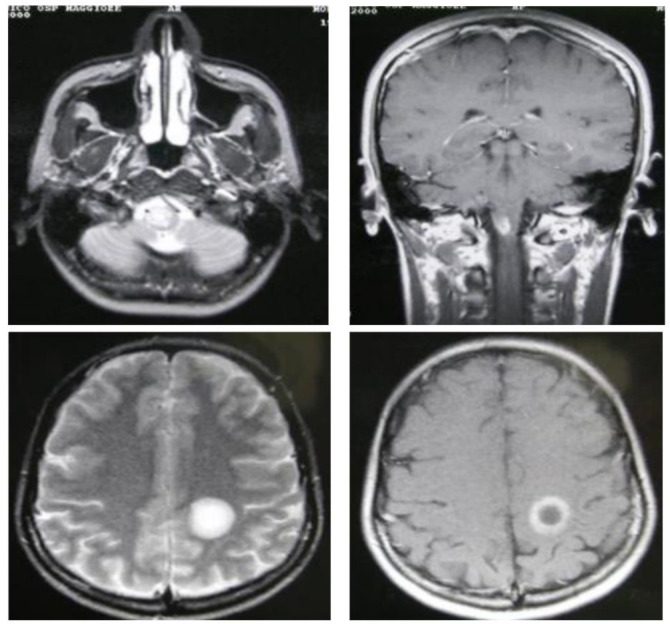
T1-weighted lesions enhanced by gadolinium.
